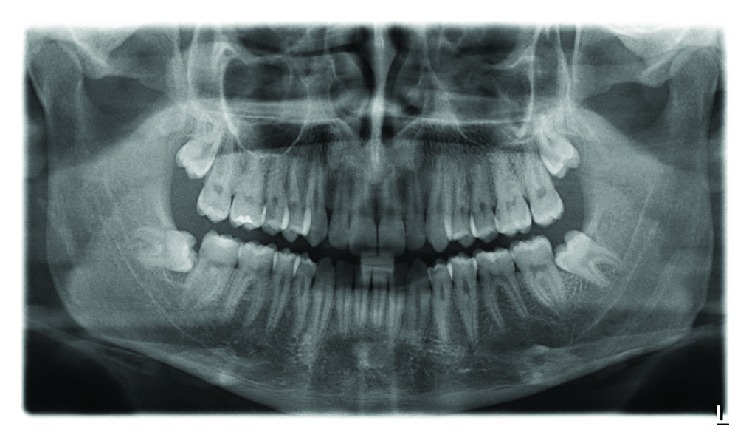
Control orthopantomography 24 months after surgery. Note the complete recovery of the lesion.
